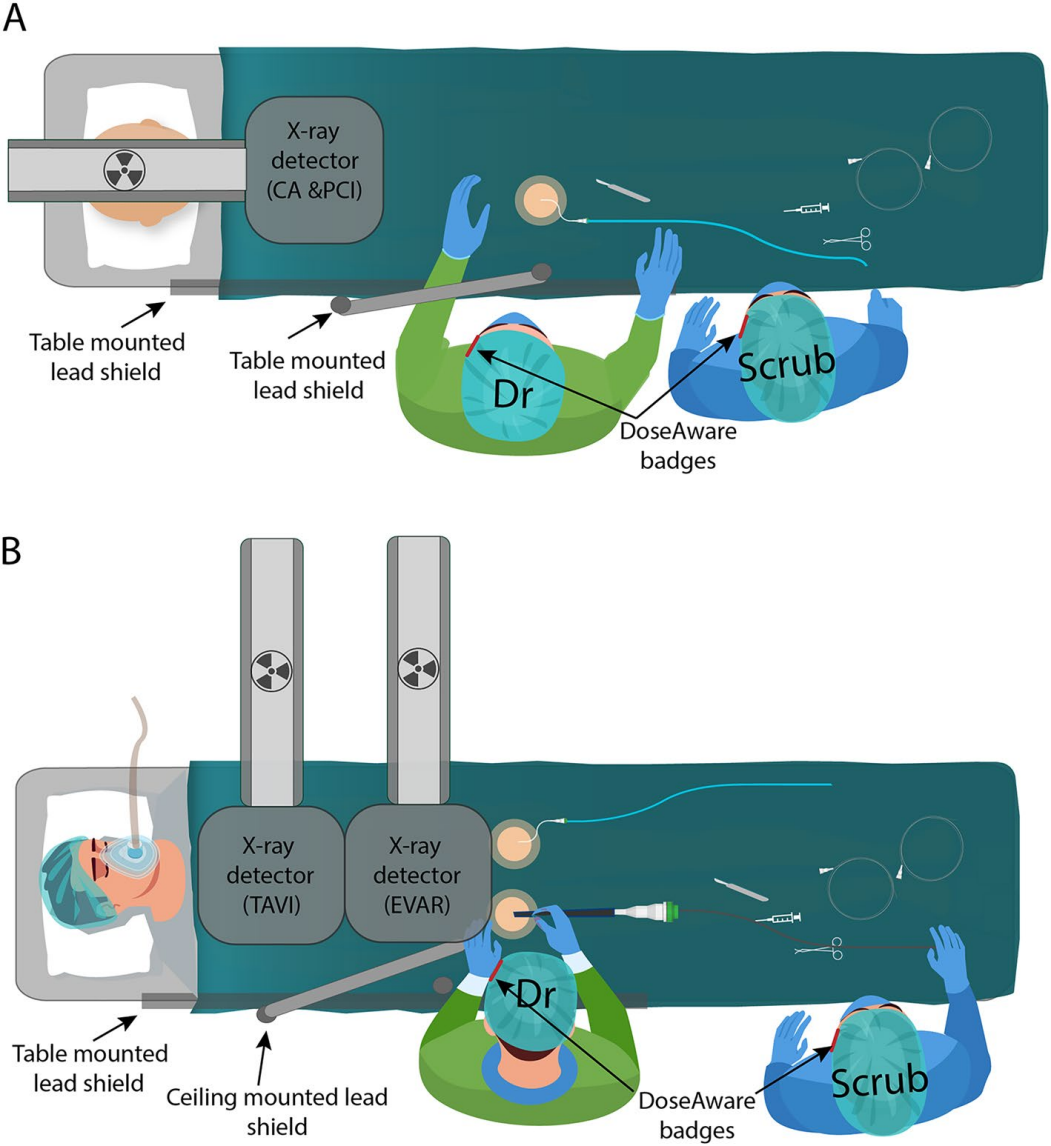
Demonstration of common staff positions in relation to the x-ray detector during CA and PCI (A), and TAVI and EVAR procedures (B). Location of the lead shielding and DoseAware badges are included. *Note—the x-ray tube is located under the patient table.
